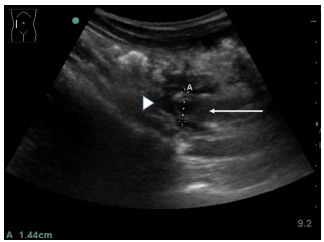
Point-of-care ultrasonography of the abdomen over the right hypochondrium demonstrated a dilated appendix (arrow) measuring 14.4 millimeters (A) with a surrounding rim of complex hypoechoic fluid (arrowhead), suggesting perforation.
